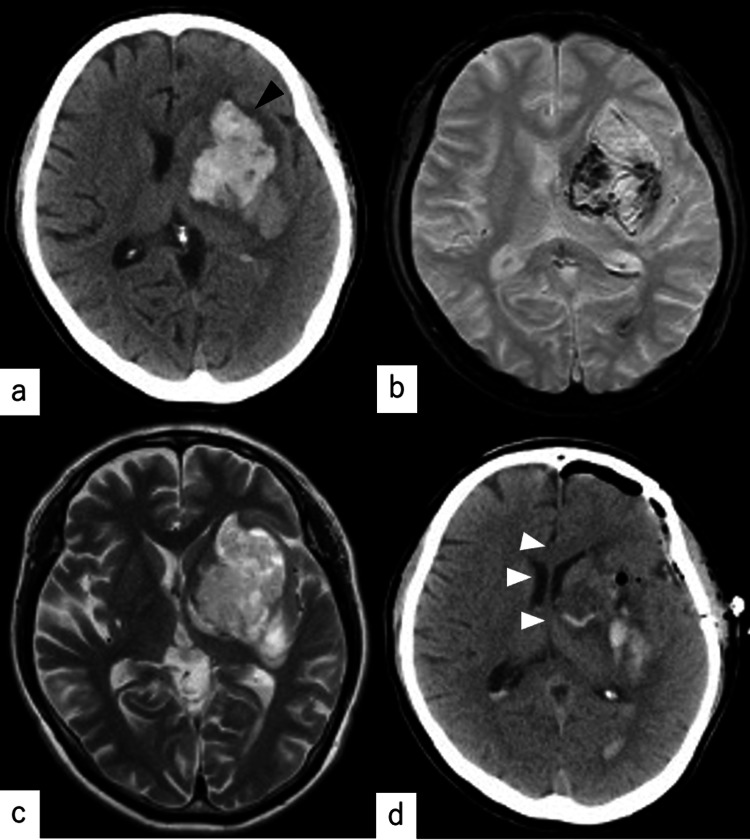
(a) Initial computed tomography (CT) in case 2: hemorrhage in the left basal ganglia (measuring 6.7 × 4.4 × 3 cm in diameter with a volume of 44.2 mL) (black arrow). (b-c) Magnetic resonance imaging (MRI): conventional T2-weighted imaging and T2-weighted imaging with a gradient-echo MRI sequence did not reveal the cavernous malformation. (d) Postoperative CT: the lesion was almost completely removed and there was a reduction in the midline shift (white arrow).
